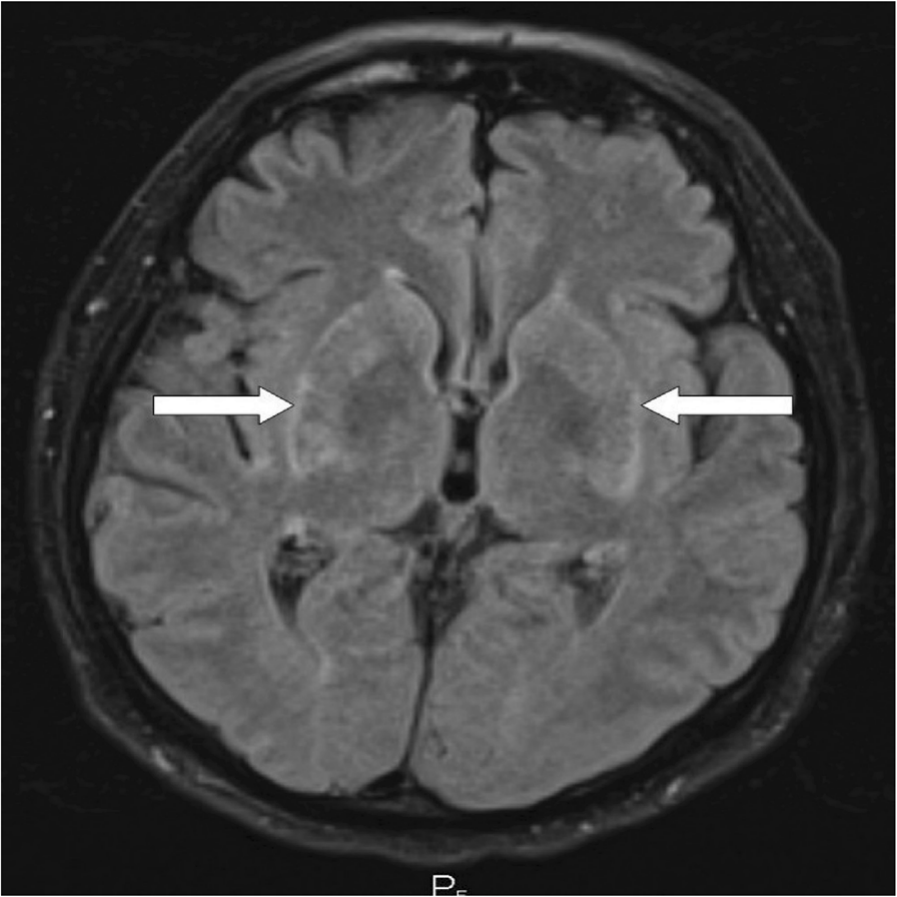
Susceptibility-weighted imaging magnetic resonance imaging of the brain showing (see arrows) an areaof inflammatory increased signal within the basal ganglia (August 2016)
